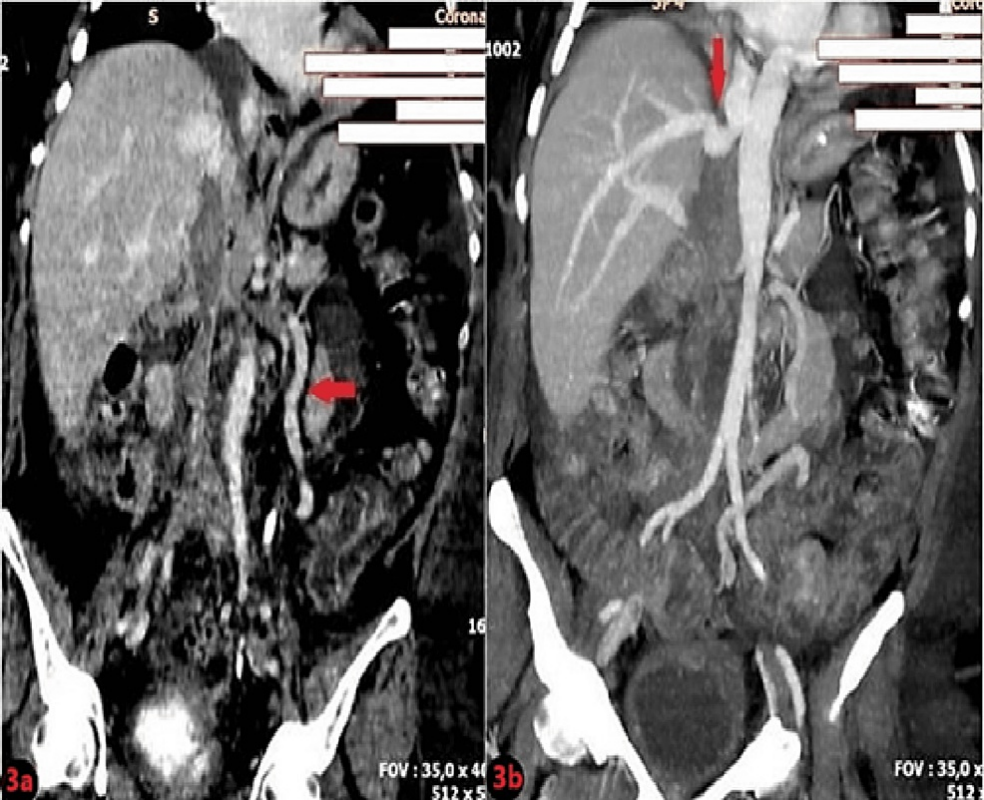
Postoperative abdominal and pelvic CT scan showing: a resection of the right kidney and tumor thrombus. The left renal vein was ligated and the spermatic vein (arrow) is left in place for the left kidney blood outflow. The inferior vena cava (IVC) is ligated below the level of the renal veins and the suprahepatic IVC is at the level of the junction with the hepatic veins (arrow). CT: computed tomography
